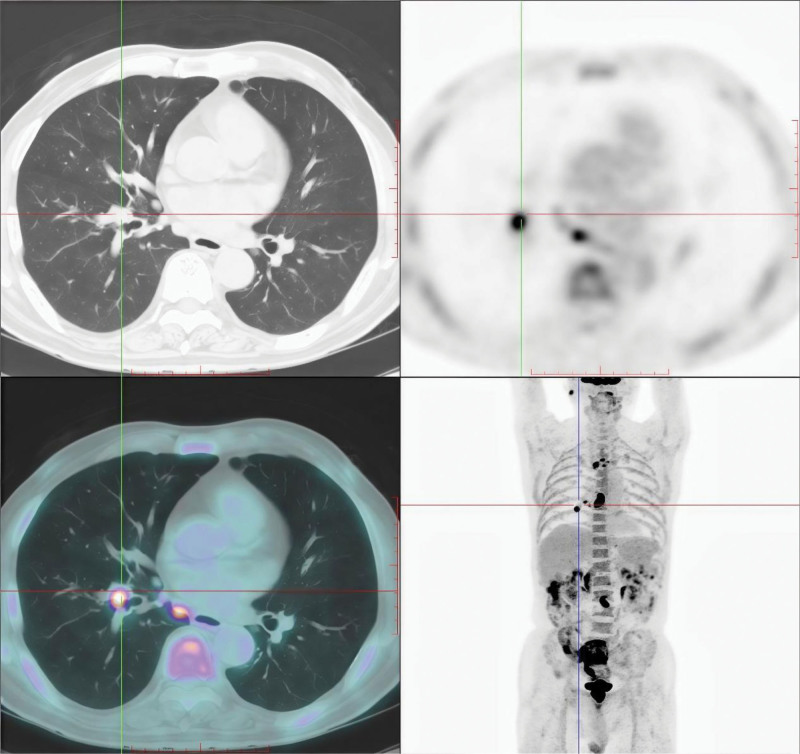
Metastatic lymphadenopathy with hypermetabolic activity in the mediastinum and right hilum PET-CT showing fluorodeoxyglucose-avid lesion in the right lower lung. PET-CT = positron emission tomography – computed tomography.
